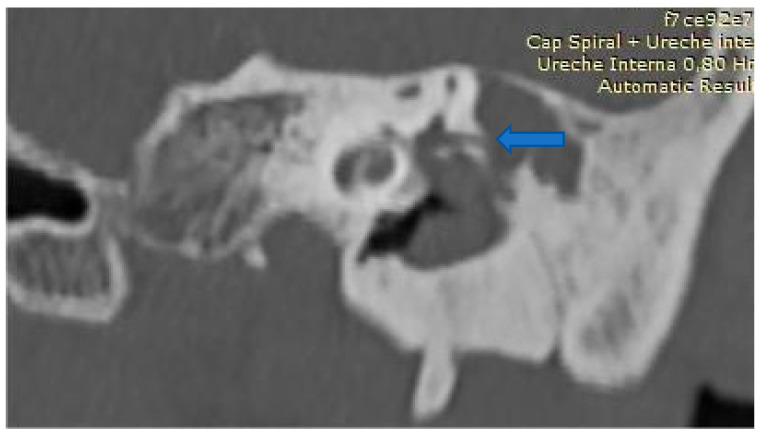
Preoperative HRCT coronal view that exposes LSCF bone erosion and presence of the cholesteatoma in the antrum and middle ear. (blue arrow fistulae).
